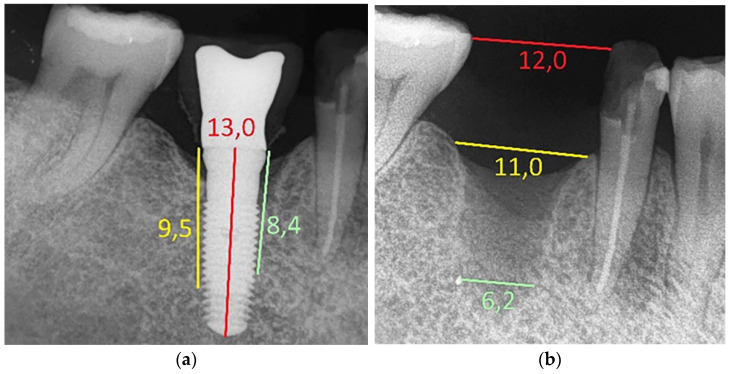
(a) Radiograph of a compromised implant positioned in the right first molar region. Although less evident on the X-ray than in case 1, peri-implantitis is more severe. In fact, the depths are 8.4 mm mesially and 9.5 mm distally, measured from the crestal level and calibrated on the actual length of the implant (13 mm). It is probable that the original positioning of this implant was subcrestal. At the time of visit, bleeding on probing and inflammation of the gingiva around the crown were noted. (b) After implant removal, the width of the defect is evident. Calibrated on the real distance between the teeth, the size of the bone defect was 11.0 mm mesio-distally at crestal level and 6.2 mm at the middle and apical part.
